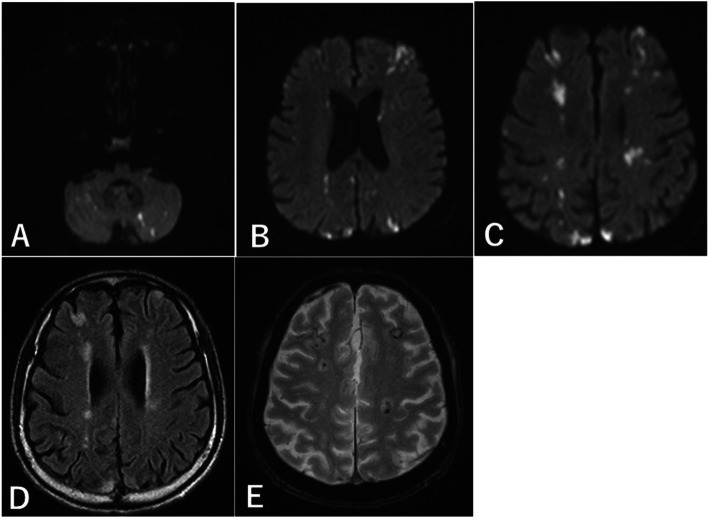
MRI images. a-c Axial diffusion-weighted image and d fluid-attenuated inversion recovery of MRI show infarctions in the bilateral watershed area of the middle cerebral artery and the cerebellar hemispheres. e Follow-up susceptibility-weighted imaging on day 15 shows the increasing microbleeds in infarcts. MRI, magnetic resonance imaging
